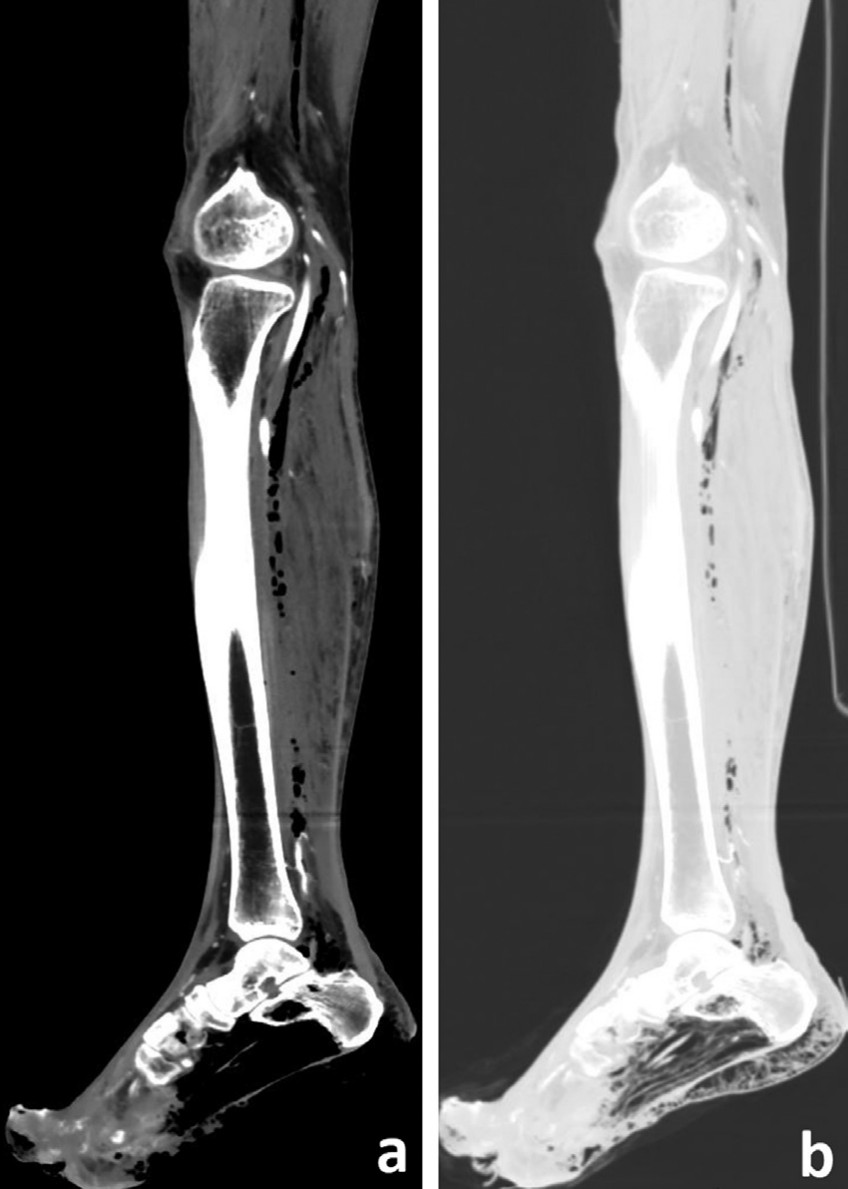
(A) Sagittal CT images of right leg and foot show presence of gas in calcaneus and sub-cutaneous planes of foot with extension of gas to deep intermuscular compartment of left lower leg superiorly up to distal thigh. Intra-osseous gas in calcaneus with extension to soft tissue supports the diagnosis of “Emphysematous osteomyelitis.” (B) Sagittal CT image in lung window supports the findings described above with black areas representing gas.
